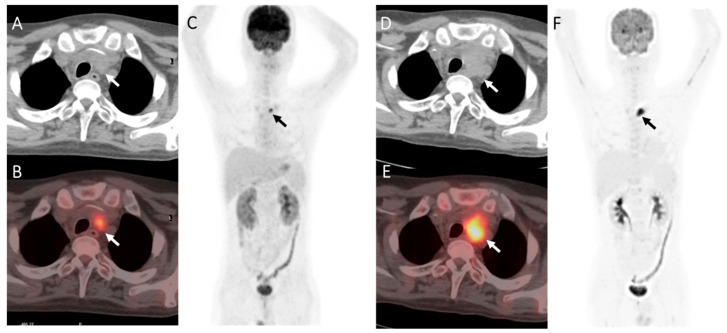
A case of T-Lymphoblastic lymphoma of the mediastinum in a 30-year-old male. The patient was treated with chemotherapy and achieved complete remission. The patient then received allogeneic SCT and underwent ^18^F-FDG PET-CT 5 months after transplantation for assessment. (A–C): PET showed an FDG-avid lesion in the upper mediastinum (arrow), about 1.7 * 1.2 cm^2^ in size, SUVmax7.61 (DS = 5). (D–F): A close follow-up PET imaging was carried out 1 months later with no treatment. It showed the upper mediastinum lesion (arrow) increased both in size (2.9 * 2.2 cm^2^) and ^18^F-FDG uptake (SUVmax10.85). Finally, it was clinically determined as a relapse.
